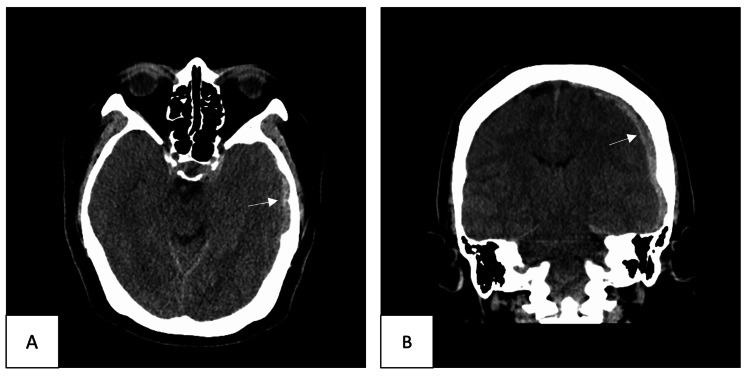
Axial (A) and coronal (B) brain CT images showed a large left acute subdural hematoma with a mild midline shift without any evidence of subarachnoid bleeding
